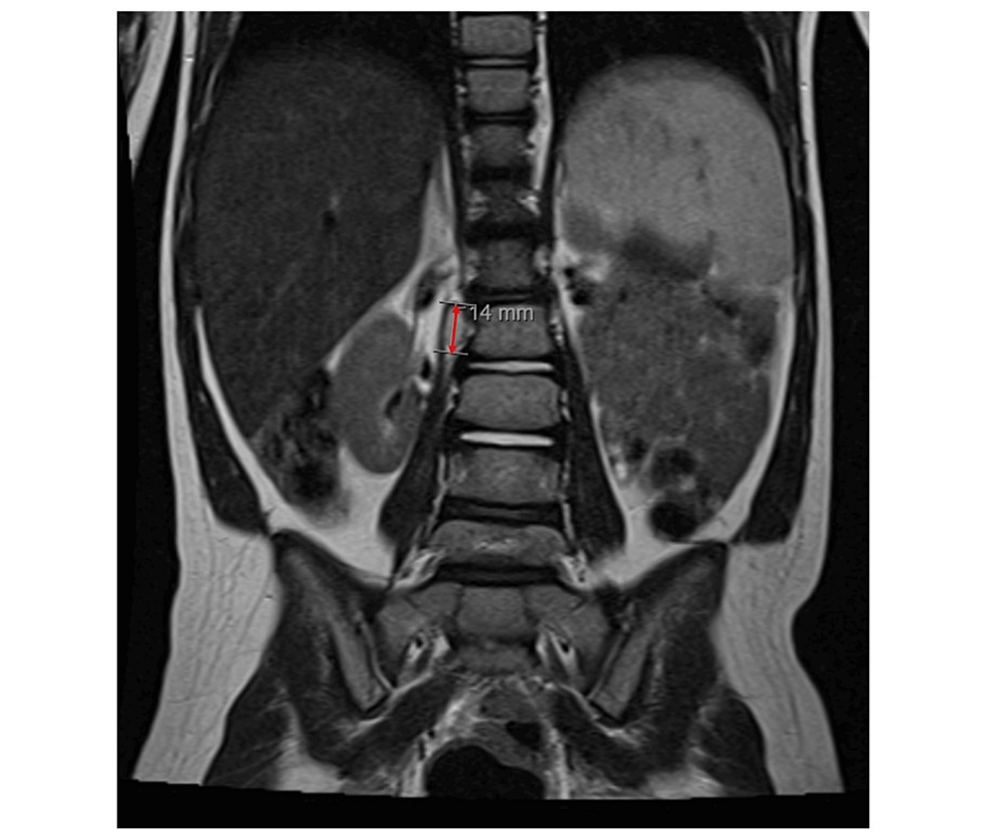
Coronal view of the dorsolumbar follow-up MRI six months after surgery. Almost total removal of the right paravertebral component of the lesion, with only a small right lateral paravertebral formation at the level of the L2 body, suggesting a small residual tumor, at the anterior and internal end of the previously observed tumor. It measures about 12 x 6 x 18 mm (vertical x transversal x anteroposterior). Resolution of the mass effect on the spinal cord.
